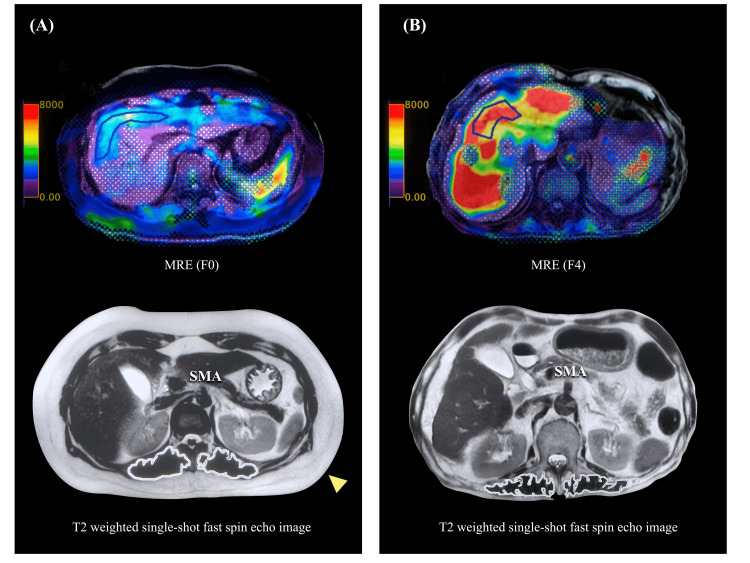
Magnetic resonance imaging. (A) Female case of CLD (F0): the upper panel shows an MRE image, and the lower panel shows a T2-weighted single-shot fast spin-echo image of the origin of the superior mesenteric artery. The white contours correspond to the left and right paraspinal muscle areas (iliopsoas, dorsalis longus, spinal cord, multifidus, and quadratus lumborum). Subcutaneous fat area (yellow arrows) was also measured in this study. (B) Male case in cirrhotic stage (F4); PSMI and fat mass decreased and diagnosed as sarcopenia and adipopenia. CLD: chronic liver disease; MRE: magnetic resonance elastography
